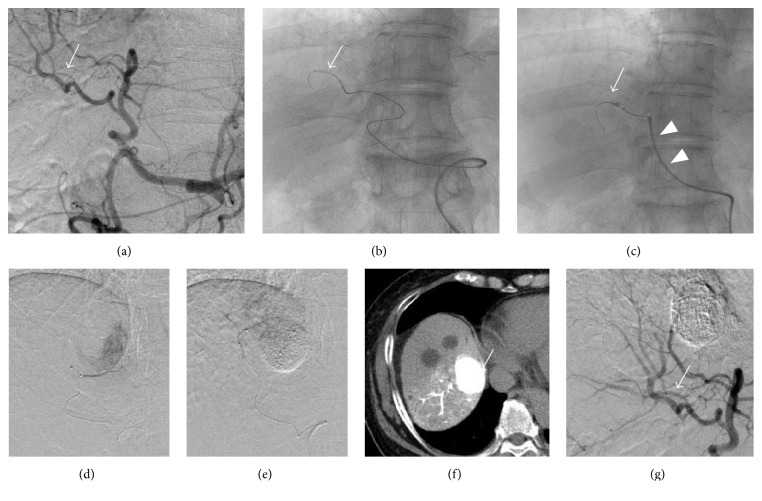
((a) and (b)) A 1.8 Fr tip coaxial microballoon catheter was inserted into a branch in segment five (arrow) that was unrelated to the feeding arteries of the tumor in segment eight. (c) The microballoon was inflated in the vessel (arrow) and the parent catheter was advanced into the right hepatic artery (arrowheads). ((d), (e), and (f)) Selective transarterial chemoembolization using miriplatin was performed and dense lipiodol deposition in the tumor was visible in the postprocedure computed tomography images. (g) Including the vessel in which the microballoon was inflated (arrow), no vascular complications were confirmed in the postprocedure angiogram.
